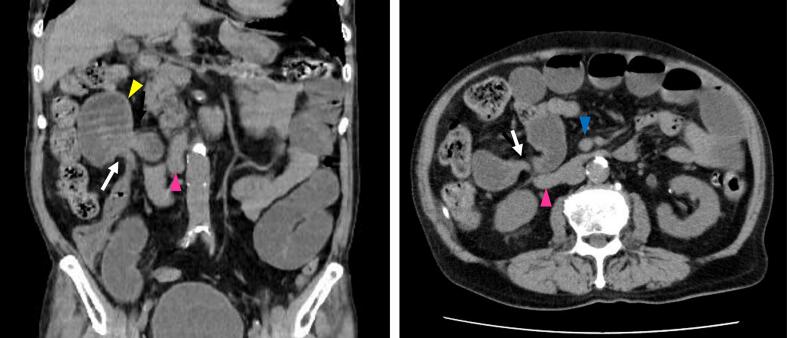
The CT scan demonstrates dilatation of the small bowel and identifies the site of intestinal obstruction (white arrow) with closed-loop (yellow arrowhead) located on the right side of the superior mesenteric vein (blue arrowhead) and anterior to the third portion of the duodenum (pink arrowhead). (For interpretation of the references to colour in this figure legend, the reader is referred to the web version of this article.)
